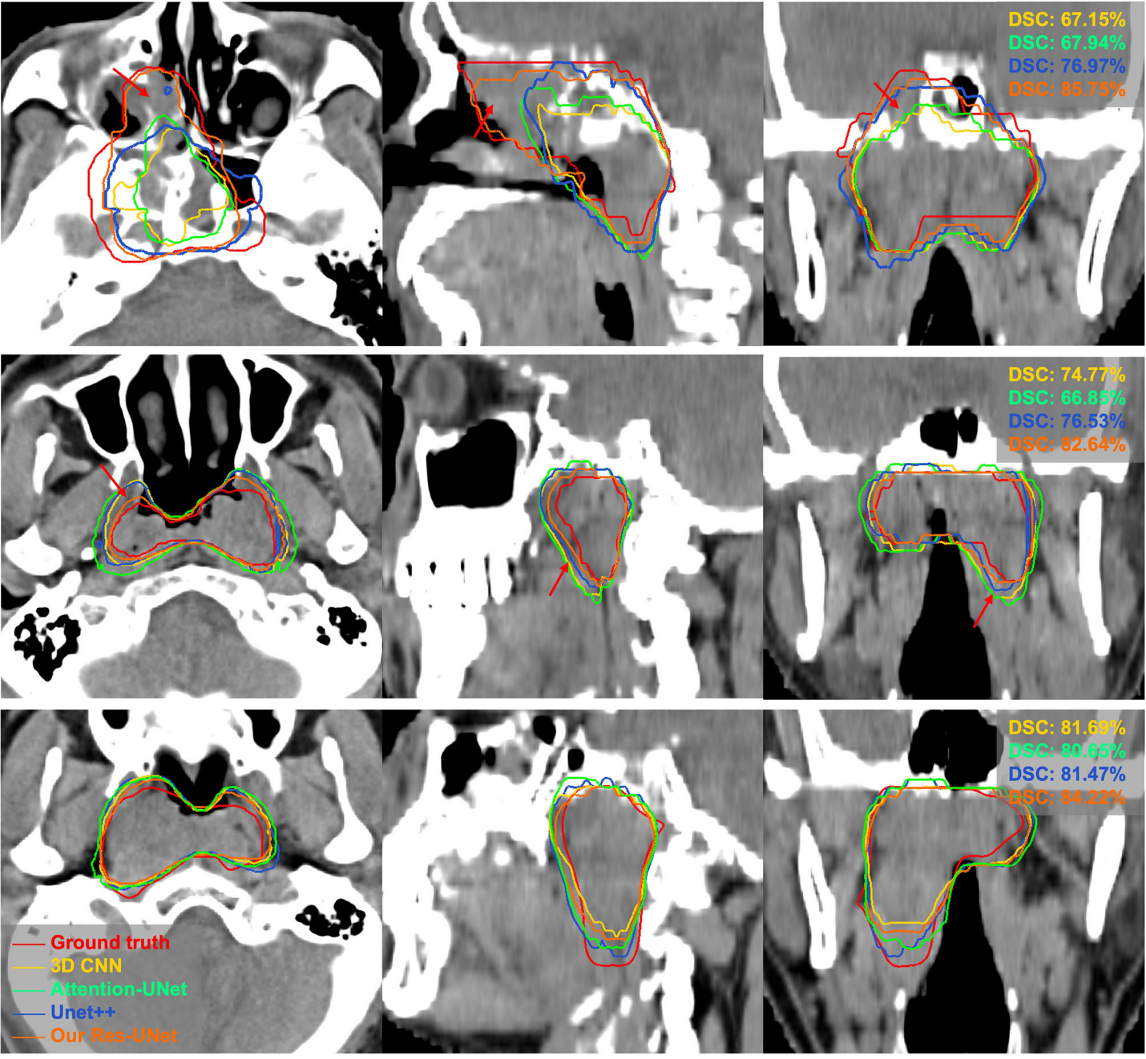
Visual comparison of different networks for GTV segmentation. The red arrows denote false positives and poorly segmented areas. Note that these results derived from the models trained on pCT and CE-CT data are shown in pCT.
